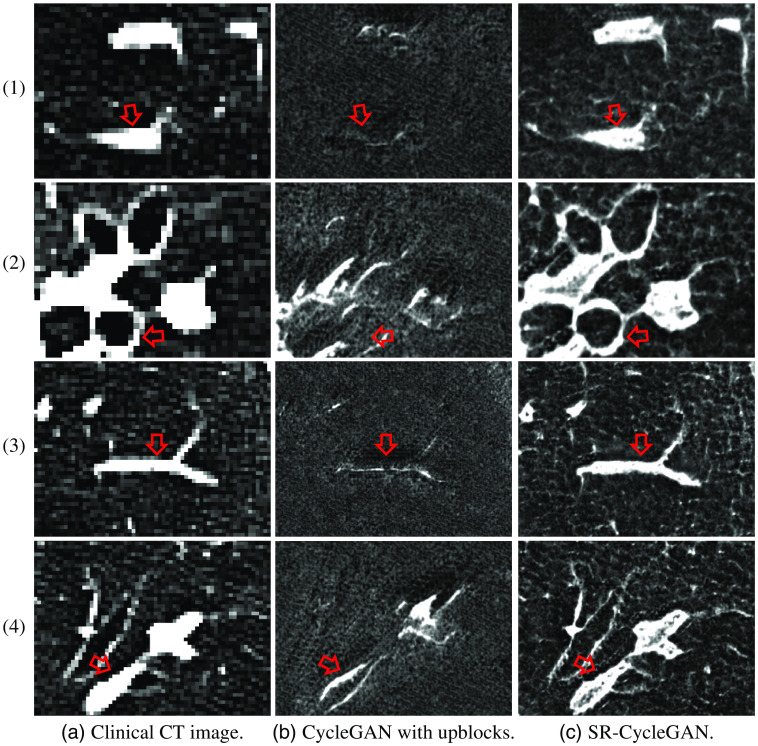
SR results of clinical CT images from one case. Rows (1) and (3) are images cropped from blood vessels and lung field region. Rows (2) and (4) are images cropped from the bronchus and blood vessels region. Column (a) are original clinical CT images. Column (b) and (c) are results of “CycleGAN with upblocks” and our method, respectively. We can obtain that SR-CycleGAN output reliable SR results, while CycleGAN with upblocks (no MMSR loss, no network modification, only upblocks for larger output image) output results that do not have similarity with the input image. As pointed by red arrows, blood vessels and bronchus in SR images of CycleGAN with upblocks severely deformed or disappeared, while blood vessels and bronchus in SR-CycleGAN’s SR images have sharp edges and same shape as in LR images.
